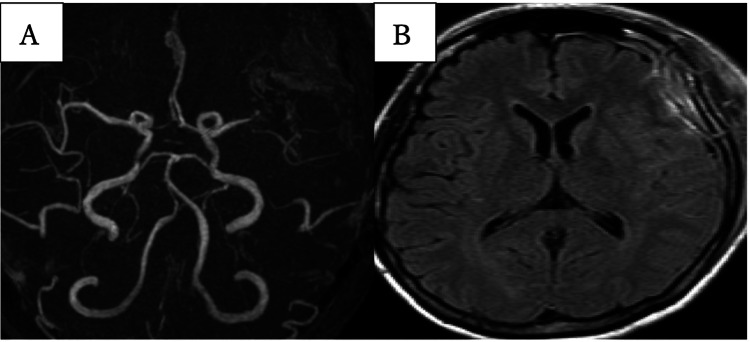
MRA and FLAIR image on postoperative day 7 (A) Poor visualization of the left MCA; (B) Signal changes in the left MCA lesion MRA, magnetic resonance angiography; FLAIR, fluid-attenuated inversion recovery; MCA, middle cerebral artery
